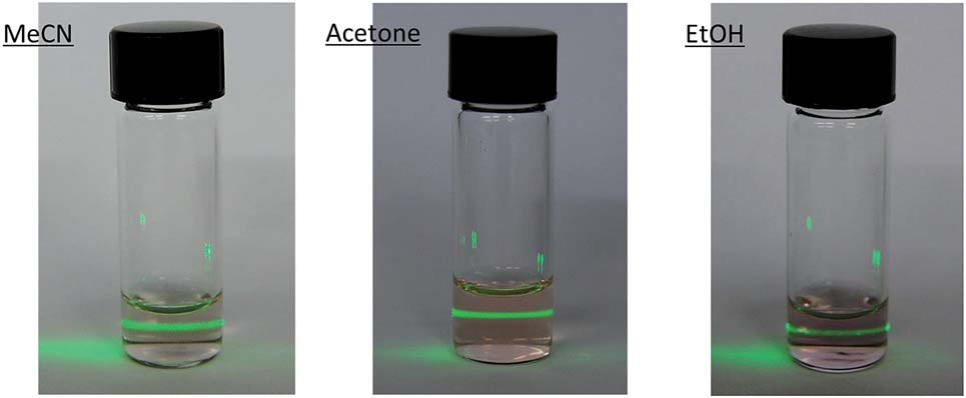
Tyndall effect of crystals of 2 after immersing in acetone, ethanol or acetonitrile (1.0 mg in 1 mL) overnight and then ultrasonicating for 1 minute.
